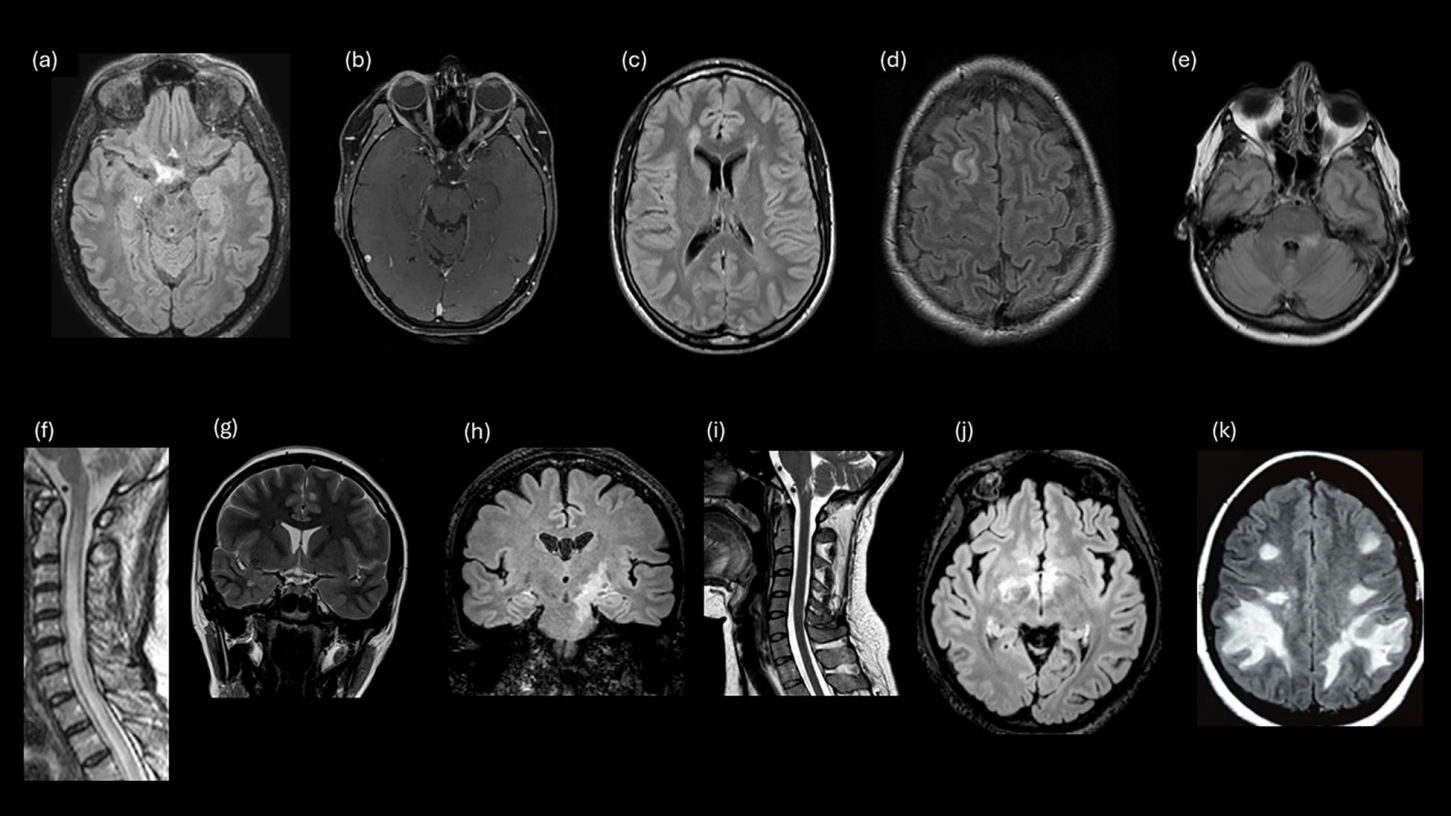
Examples of study survey images that evaluated participant knowledge for MRI lesions. (A) Lesion involving optic chiasm and posterior ON. (B) LEON (longitudinally extensive left optic neuritis). (C) Periventricular (MS). (D) Juxtacortical (MS). (E) Fluffy lesion and poorly demarcated lesion (MOGAD). (F) LETM. (G) Cortical lesion lob temporal (MS). (H) Corticospinal tract lesion. (I) Lesion involving the area postrema. (J) Hypothalamic lesion. (K) Large, confluent, bilateral subcortical or deep white matter lesions.
